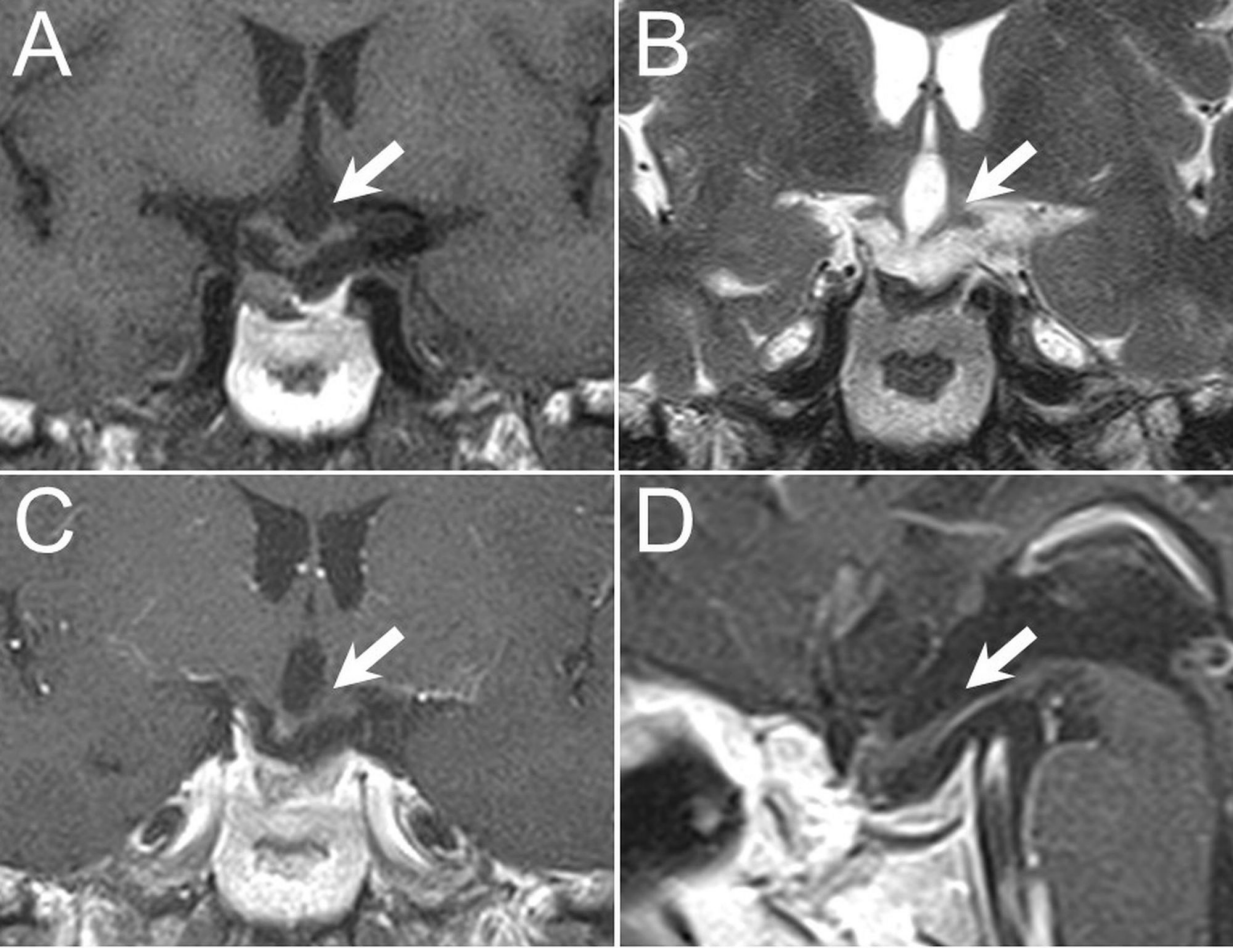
Post-operative magnetic resonance imaging (MRI) Post-operative MRI image demonstrated no residual tumor (arrows) over T1-weighted (A: coronal view), T2-weighted (B: coronal view), and gadolinium-enhanced T1-weighted images (C: coronal view, D: sagittal view).
